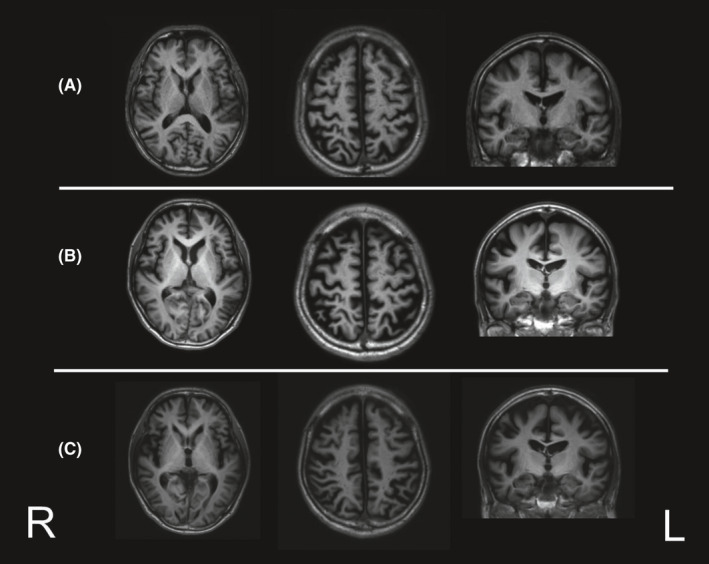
Magnetic resonance images (A) at baseline, (B) 18 months later, and (C) 3 years later. The bilateral superior parietal lobes show slight atrophy for his age, suggesting Alzheimer's disease pattern
